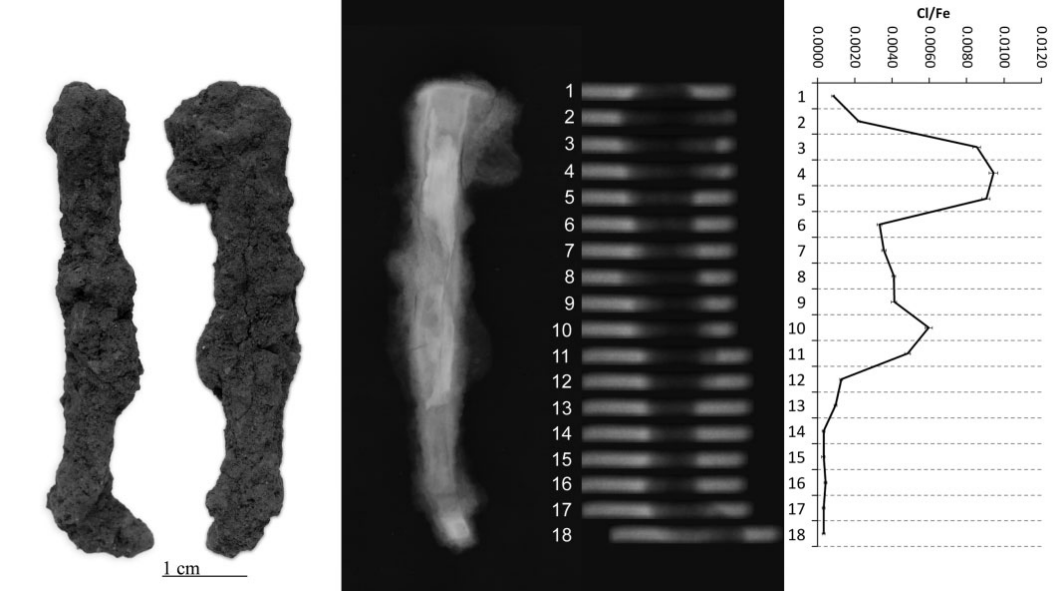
Left to right: a photograph, X-ray radiograph, neutron radiograph and the PGAI data for CPF08_064, using a 3 × 20 mm collimator.
